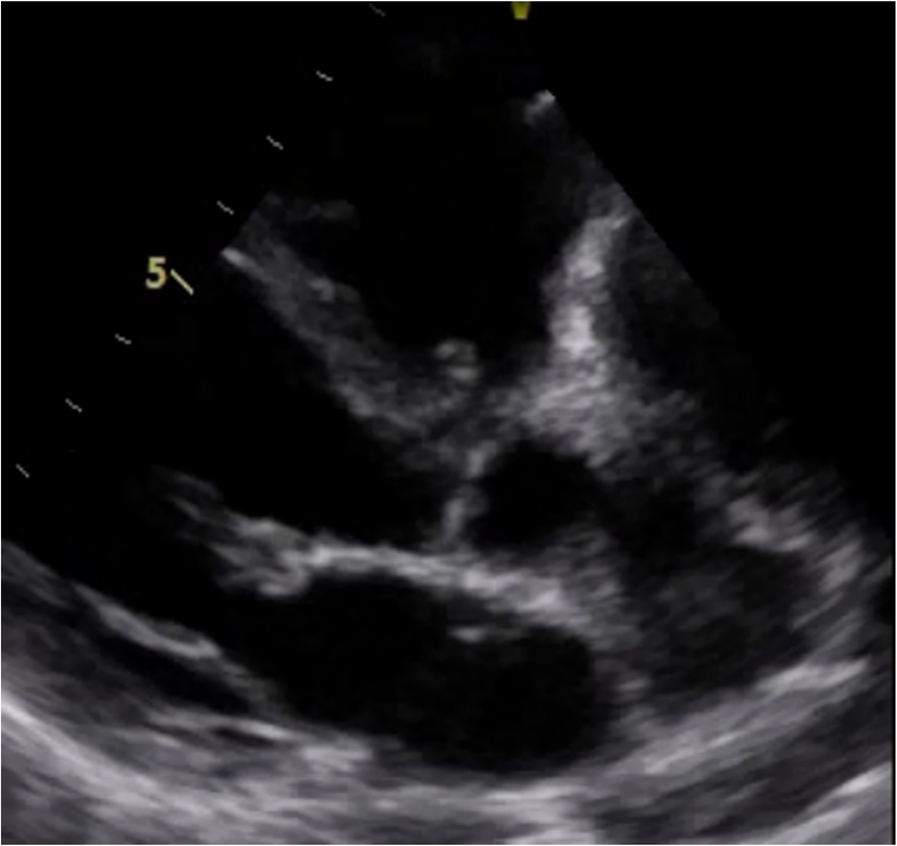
Parasternal long-axis view showed thickened left-sided valves
